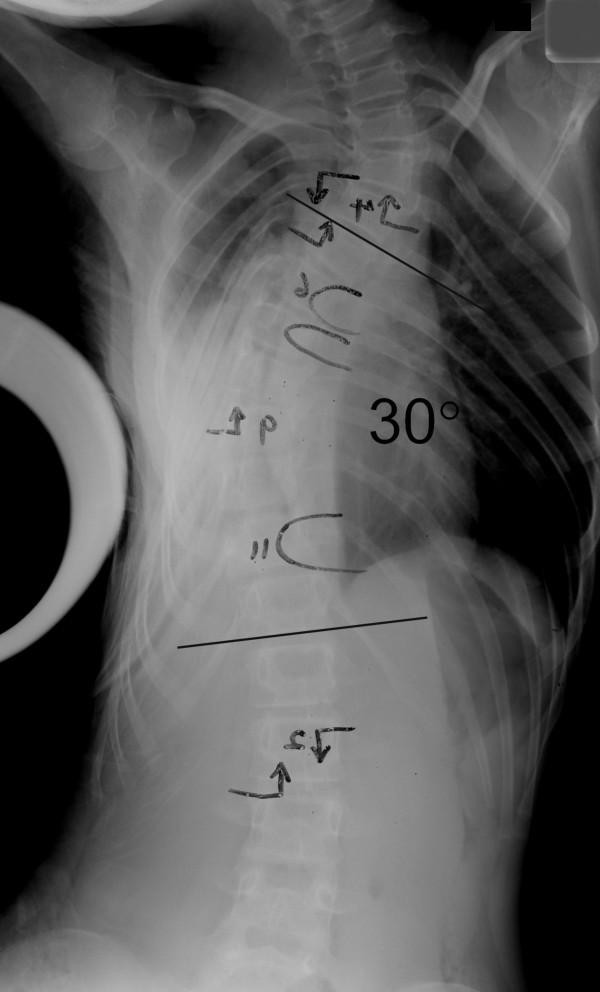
Fulcrum bending radiograph after anterior release.
